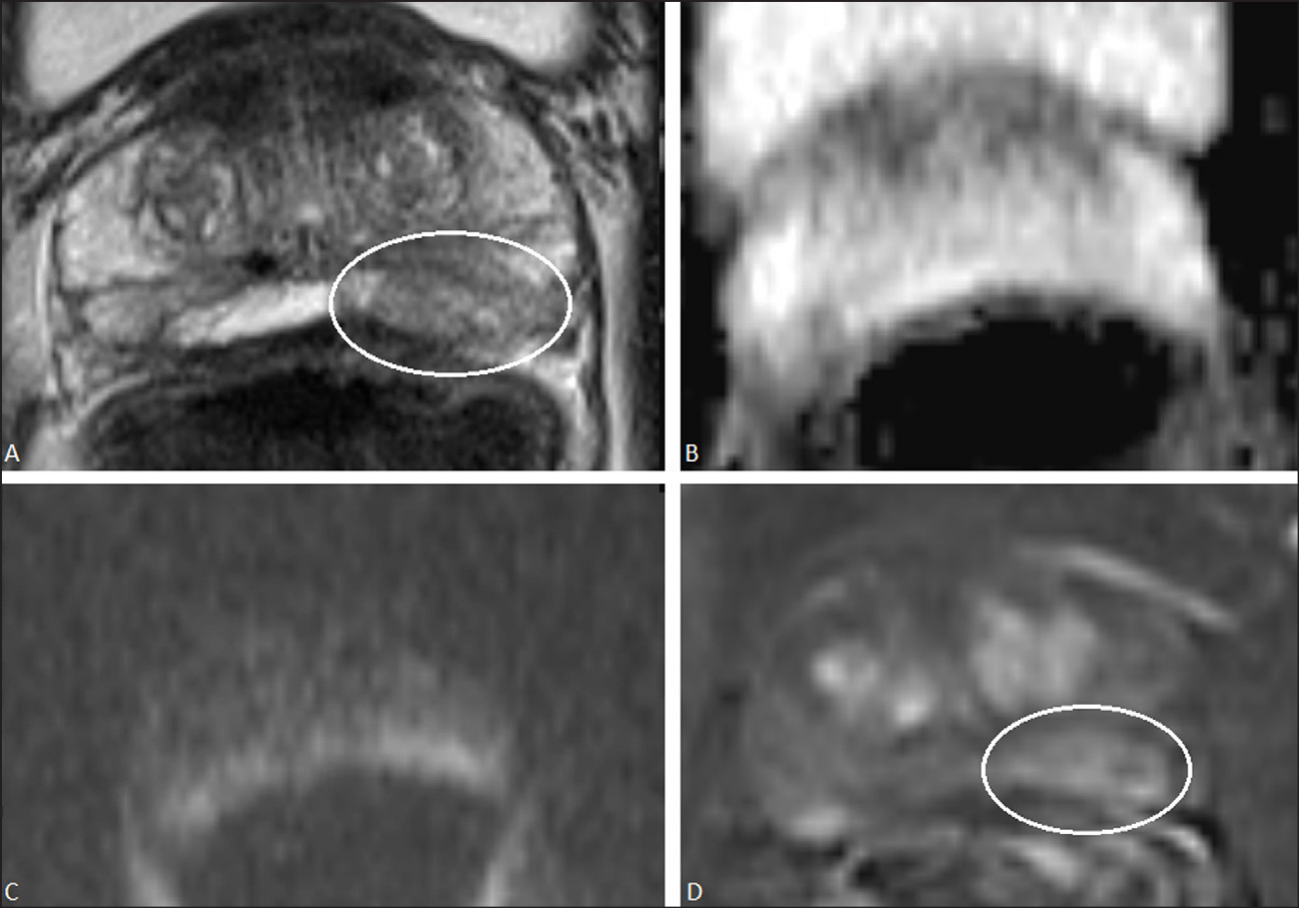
A 63-year-old man with a PSA of 6.3 μg/L. On T2-WI (a) in the PZ an ill-defined low signal intensity focus is present posteriorly on the left side in the prostate base (white oval) (PI-RADSv1 score 4; PI-RADSv2 score 4). On DWI the signal intensity on ADC is high (b) and on high-b-value image isointense/mildly hyperintense (c) (PI-RADSv1 score 1; PI-RADSv2 score 1). On DCE (4) the area shows strong contrast enhancement (white oval) (PI-RADSv1 score 4; PI-RADSv2 negative). With the PI-RADSv1 scoring system this patient was assigned an overall assessment score 4, based on a subjective impression of the findings on T2-WI and DCE. With the PI-RADSv2 scoring system, this patient was assigned an overall assessment score of 1 since DWI is the dominant modality in the PZ. A prostate biopsy followed by radical prostatectomy in this patient showed however a Gleason 3+4 prostate cancer on the left side in the PZ of the prostate base.
